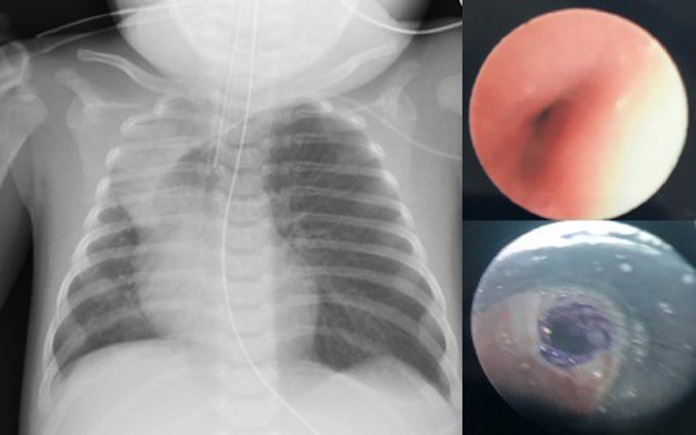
(Left) Chest X-ray taken aged 8 weeks after admission to intensive care. Marked hyperinflation of left upper lobe with collapse of right upper lobe and significant mediastinal shift to the right. (Top right) Image taken from first flexible bronchoscopy showing severe left main stem bronchomalacia. (Bottom right) Image taken immediately after first biodegradable stent insertion to left main bronchus age 13 weeks.
